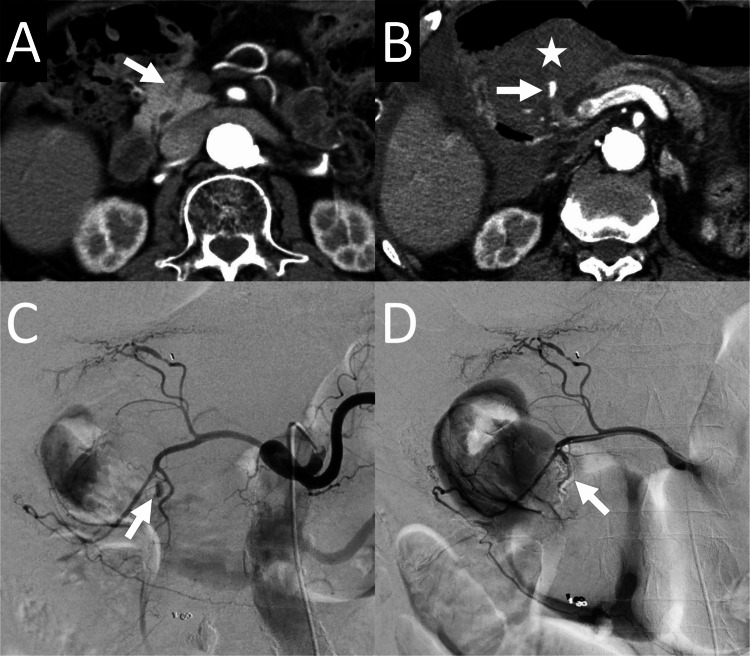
83-year-old man who underwent transcatheter arterial embolization for bleeding caused by endoscopic ultrasound-guided fine-needle aspiration (EUS-FNA) for a pancreatic head lesion (patient number 6) A: An axial arterial-phase contrast-enhanced computed tomography (CECT) image obtained before EUS-FNA reveals a slightly hypodense lesion (arrow) in the pancreatic head. B: An axial arterial-phase CECT obtained after EUS-FNA reveals contrast-media extravasation (arrow) near the pancreatic head. A hematoma (star) surrounding the extravasation is observed. C: Angiography of the celiac artery reveals a pseudoaneurysm (arrow). Although the location of the pseudoaneurysm is not clear from the image, the pseudoaneurysm was in the anterior superior pancreaticoduodenal artery (ASPDA). D: Angiography of the gastroduodenal artery after embolization of the ASPDA with N-butyl cyanoacrylate (NBCA) reveals no pseudoaneurysm opacification. NBCA cast is observed in the pseudoaneurysm and the posterior superior pancreaticoduodenal artery due to backflow (arrow).
